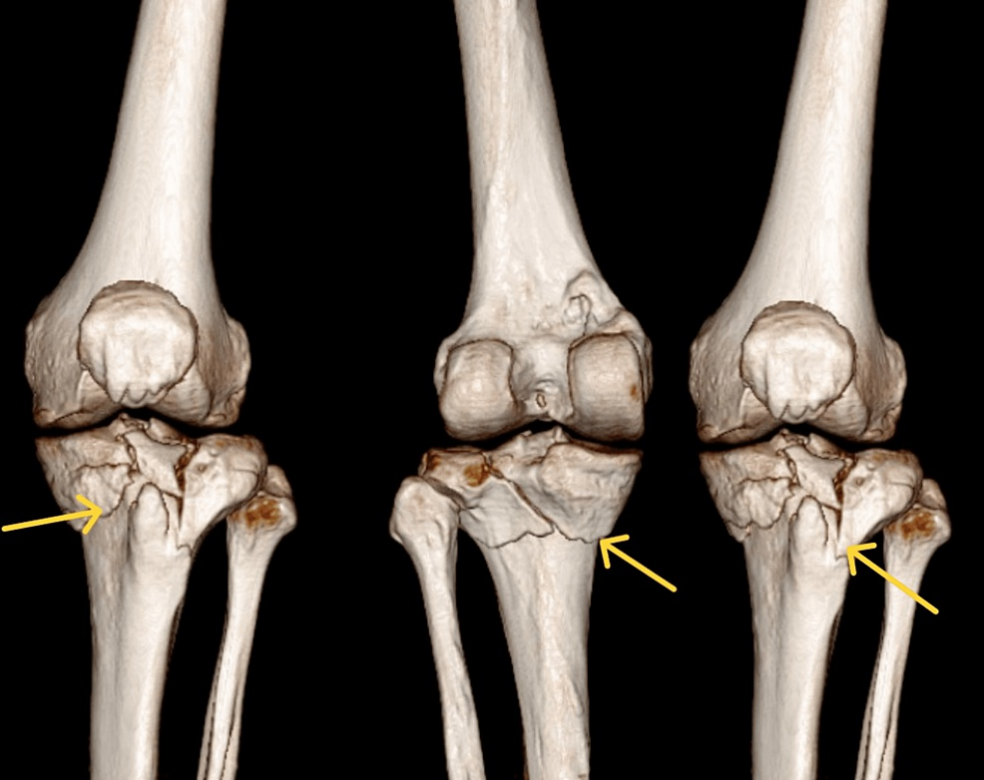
CT scan of the left knee joint
